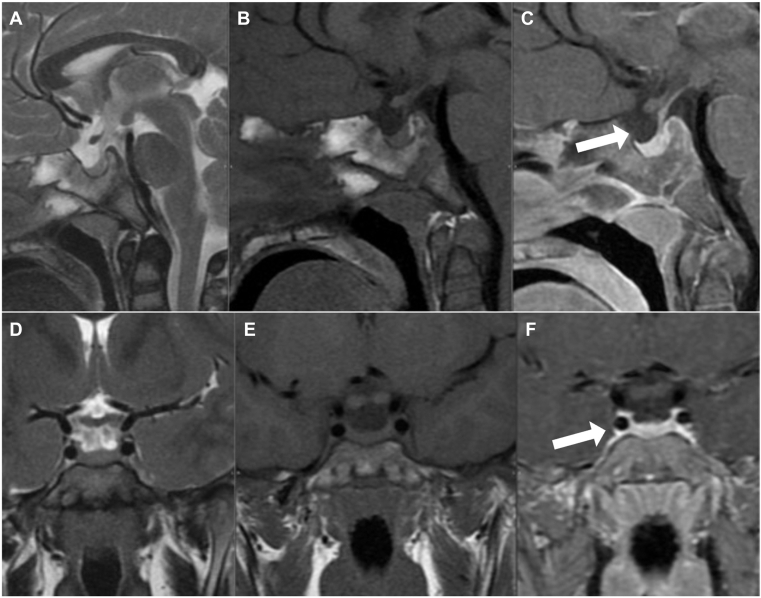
Follow-up pituitary MRI. (A–C) Sagittal T2, T1, and T1 postcontrast sequences show normal pituitary gland morphology (white arrows). (D–F) Coronal T2, T1, and T1 postcontrast dynamic sequences demonstrate a centrally located pituitary stalk without optic chiasm involvement (white arrows). MRI = magnetic resonance imaging.
